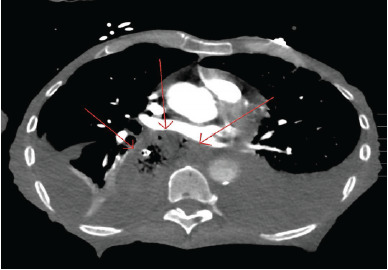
Transverse view from computed tomography angiography of the chest showing evidence of a distended esophagus with a large amount of intraluminal contents (arrows).
